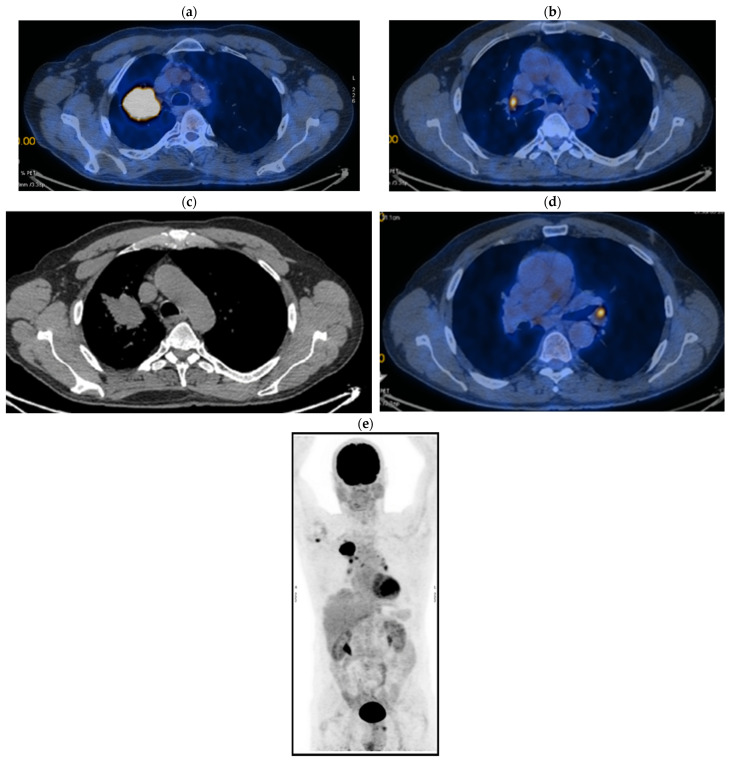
PET CT. (a) T—spiculiform LSD tumor formation of 44 × 57 × 63 mm with SUV = 12.59, with central necrosis; (b) the tumor invades the superior apical and mediastinal pleura; (c) N—metabolically active mediastinal ADP with SUV max = 7.43 located in the Ao-pulmonary window (14 × 9 mm), (d) infracarinal (12.8 mm) and bilateral hilar (right maximum 21 × 16 mm and left maximum 11 × 7 mm); (e) M—metabolically active focus with SUV = 5.6 right humeral head, which corresponds to a small interruption of the bone cortex.
